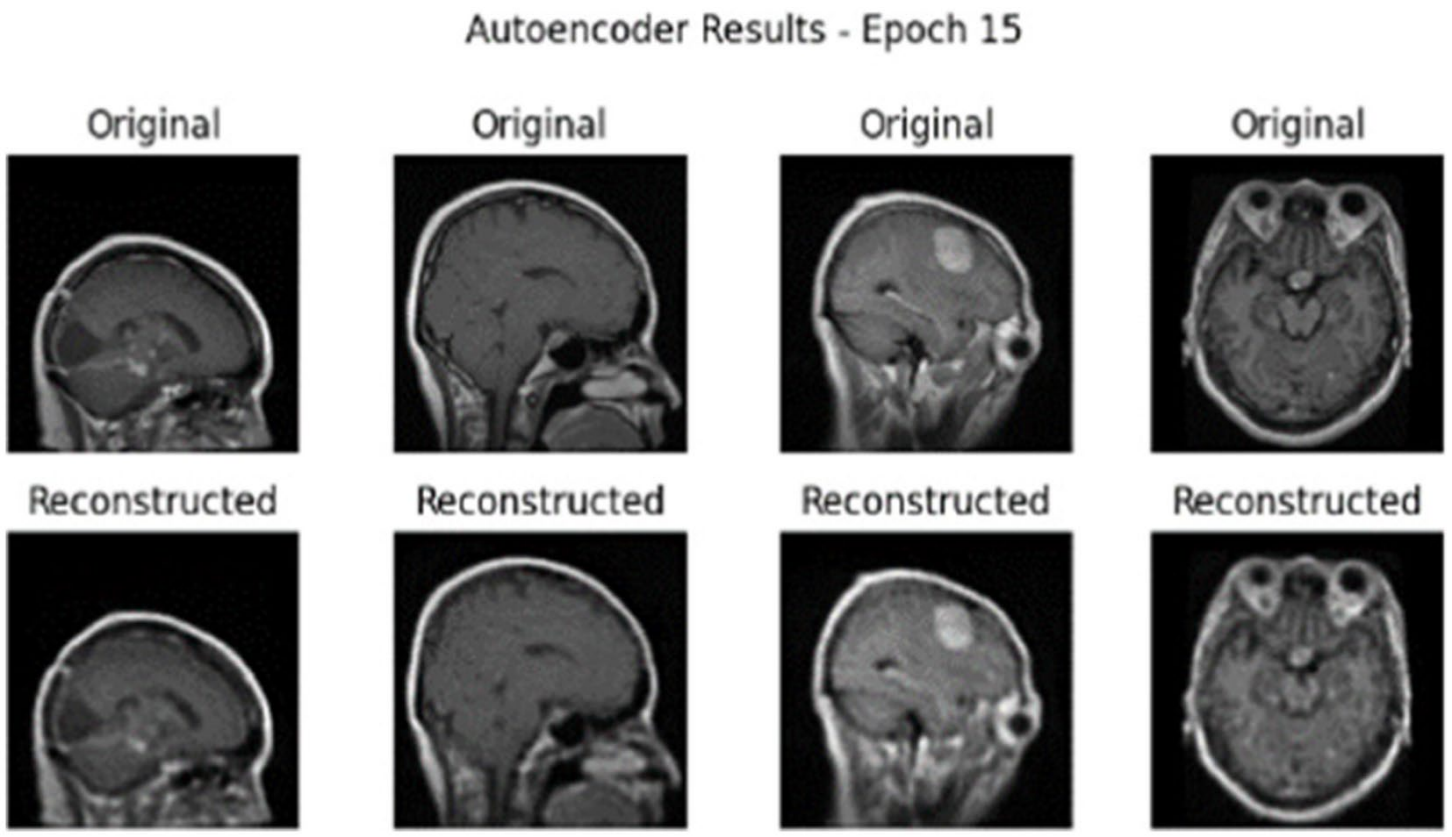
AE reconstruction results for MRI brain scans at epoch 15 on Dataset II.
